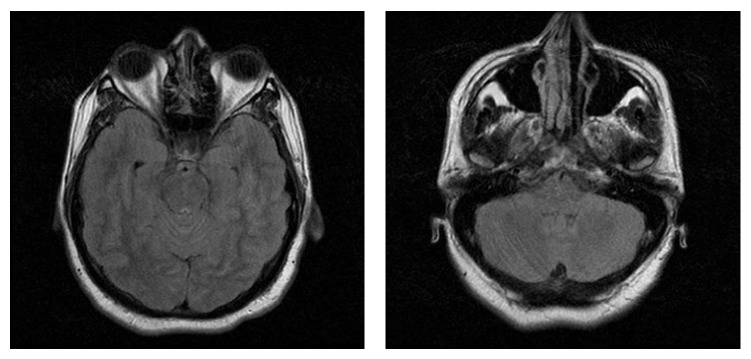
Brain MRI in FLAIR sequence, without abnormalities.
